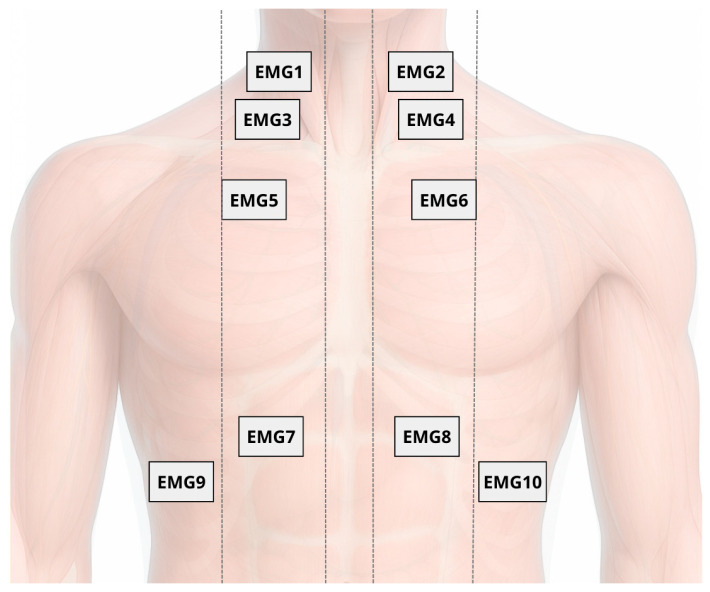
Placement of 10 surface electromyography (sEMG) electrodes on respiratory muscles: scalene (EMG1 and EMG2), sternocleidomastoid (EMG3 and EMG4), parasternal (EMG5 and EMG6), and hemidiaphragms (EMG7 to EMG10), bilaterally positioned according to SENIAM guidelines.
